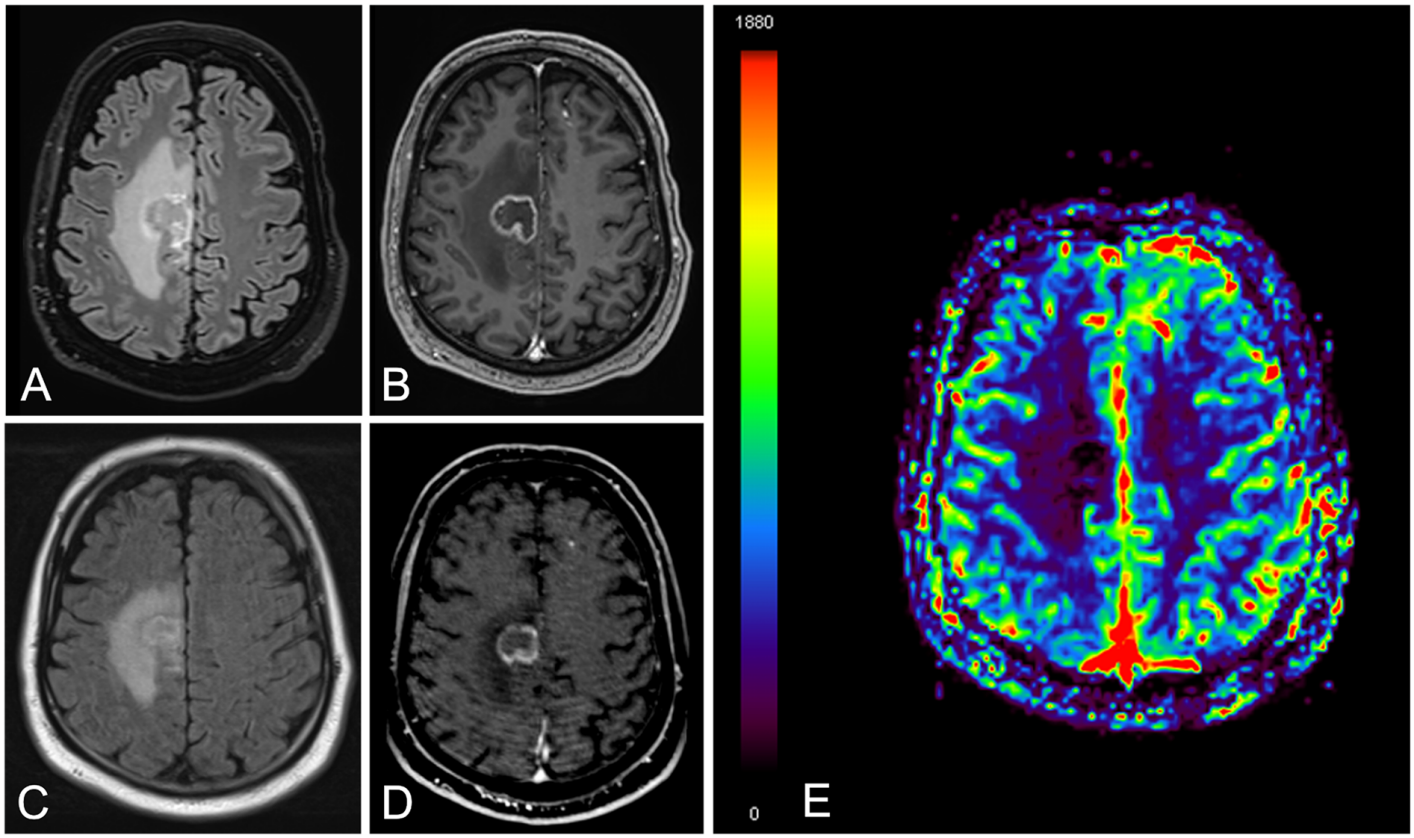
A 76-year-old male patient with brain metastasis and lung cancer. Brain MRI images obtained at eight months since stereotactic radiation therapy [(A) FLAIR and (B) contrast enhanced T1]. Follow-up MRI study at six months [(C) FLAIR, (D) contrast enhanced T1 and (E) relative cerebral blood volume map]. There is a right frontal ring enhancing lesion with peripheral vasogenic edema (A–D), showing slight reduction in size at 6 months (C,D) together with lack of increased perfusion (E) The findings were consistent with radiation necrosis.
